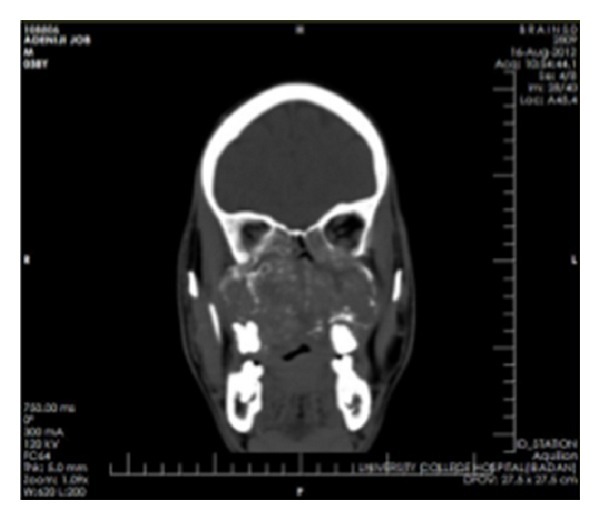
Coronal CT showing involvement of the paranasal sinuses and orbital floor.
